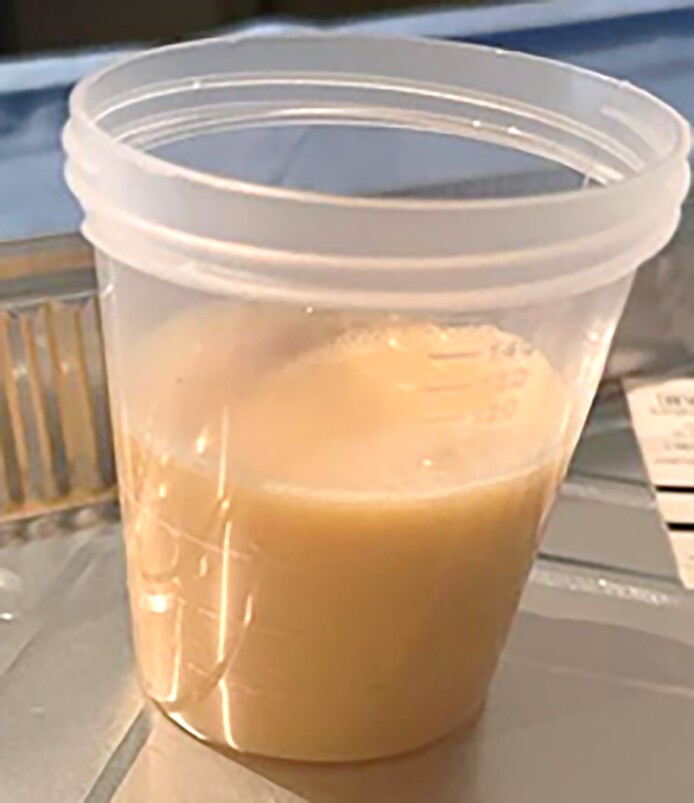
Lactescent fluid aspirated from the left breast of the 37-year-old female patient in Case 2, performed under ultrasound guidance.
